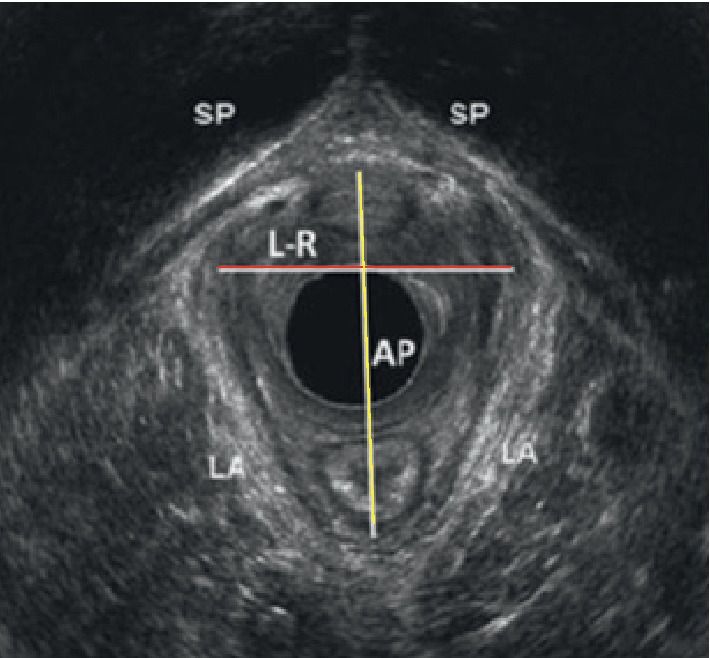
An Hiatus biometry measurement on 3D endovaginal ultrasound, intact levator ani muscle. AP, anteroposterior; L-R, left-to-right width; SP, symphysis pubis; LA, levator ani.
